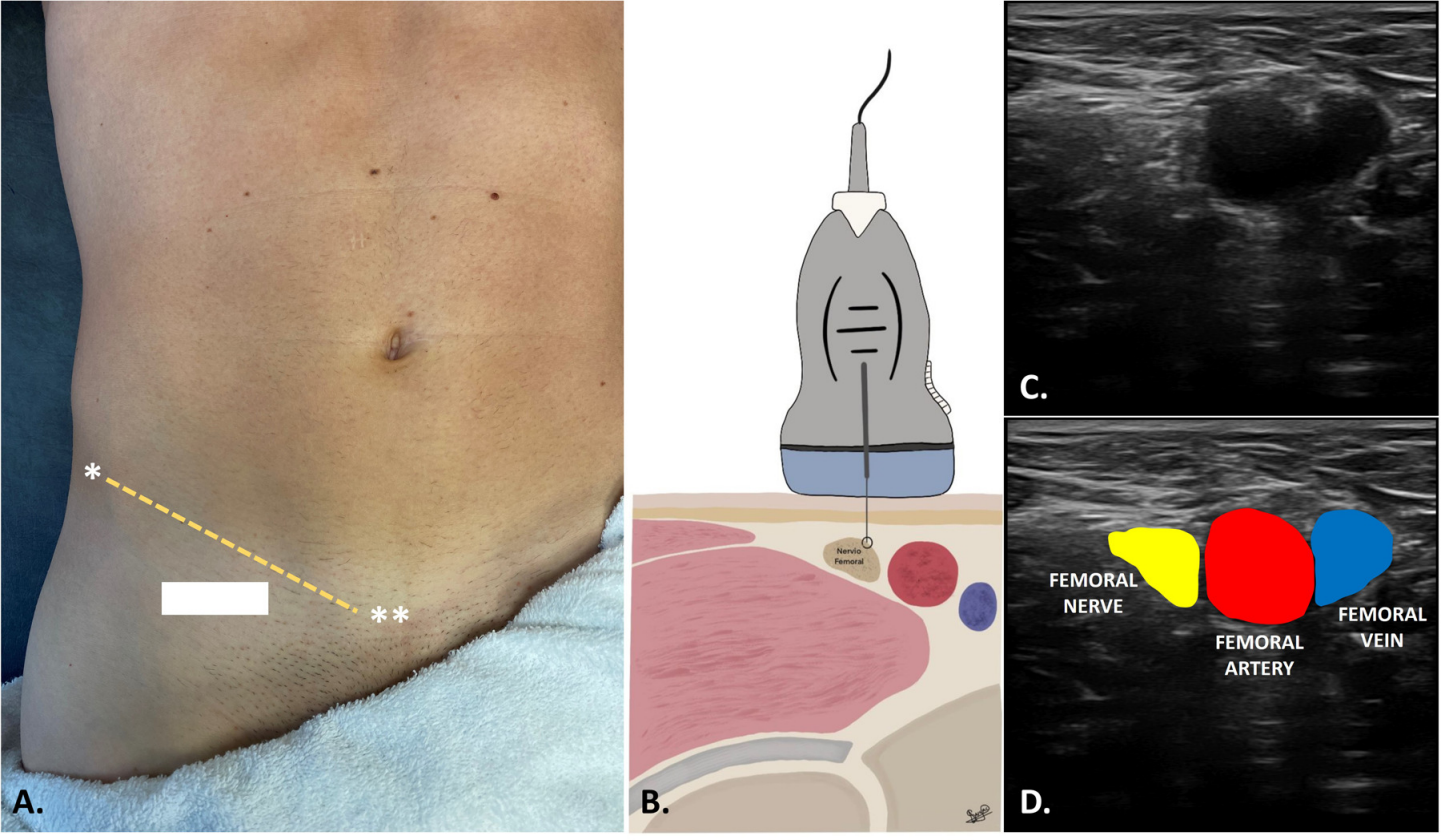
Location of the ultrasound probe and visualization of the femoral nerve for the femoral nerve invasive approach. (A) Location of the probe below the inguinal fold at the level of the pubic tubercle (), below the imaginary line between the anterior superior iliac spine (*); (B) Representation of the peripheral placement of the needle in relation to the position of the femoral nerve with respect to the femoral vascular structures (C,D). The needle entry approach was performed “out of plane” by passing the needle over the lateral edges of the perineurium (white dashed arrows showing the needle positioning) at the lateral or superior border of the nerve.
